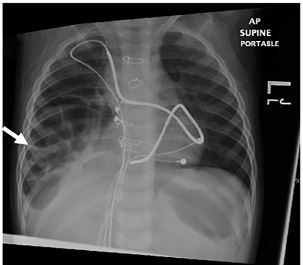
Anteroposterior chest radiograph (CXR) performed on a child who presented to the emergency department in respiratory distress; CXR demonstrated significant elevation of the right hemidiaphragm with colonic interposition and right lung base infiltrate (arrow).
